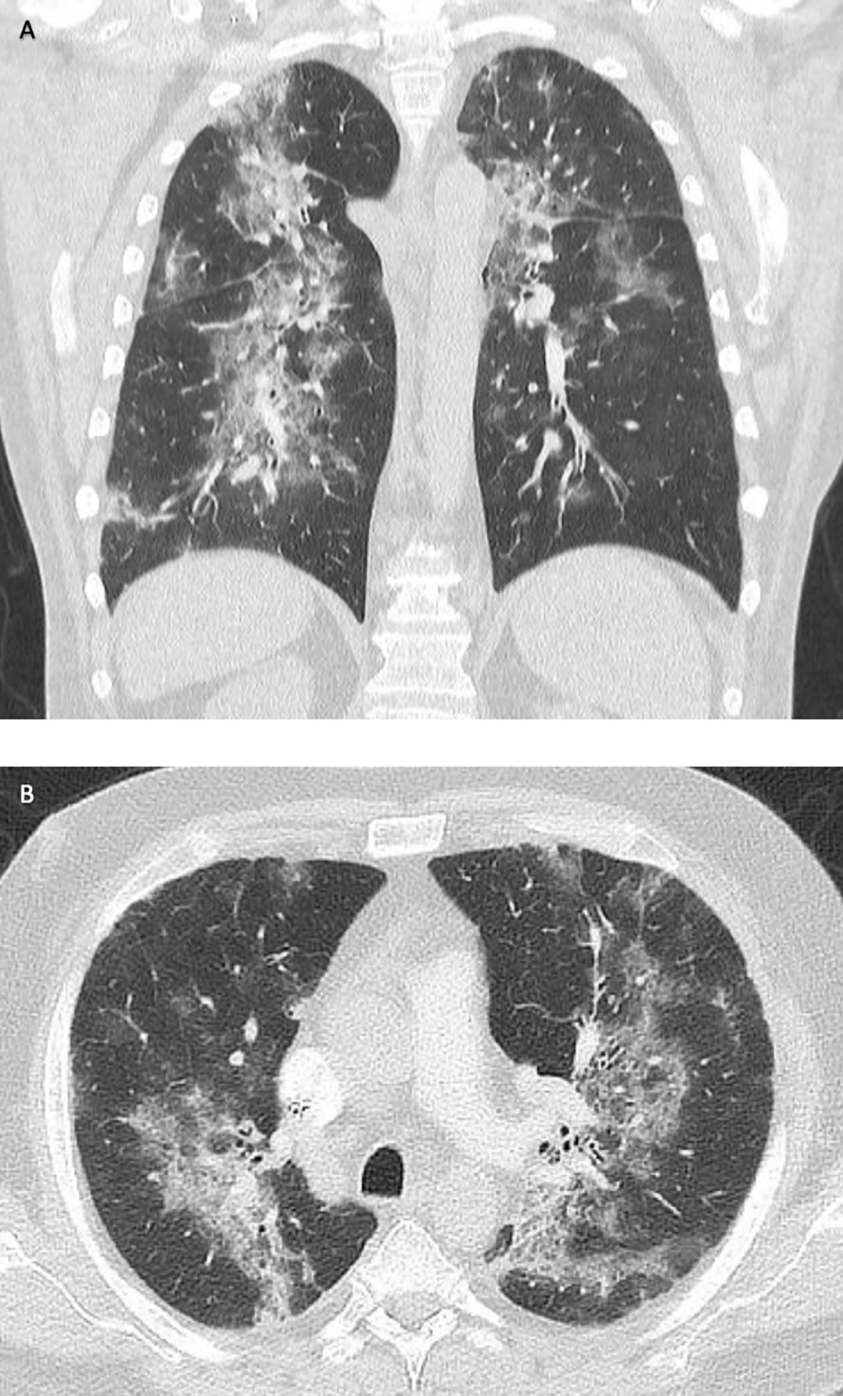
Computed tomography (CT) of the lungs in frontal (A) and transverse sections (B). Disseminated ground-glass opacities due to COVID-19 pneumonia (December 2020).
